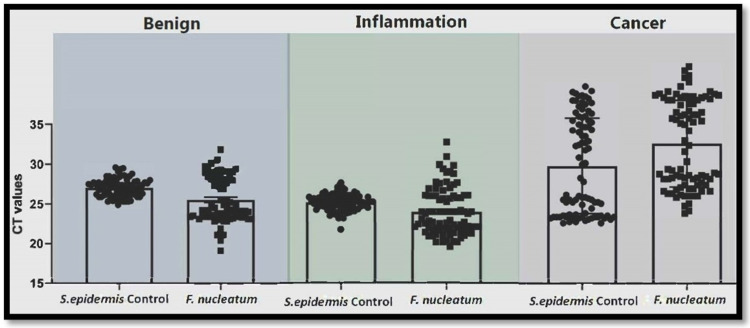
CT values of control S. epidermidis and F. nucleatum of all three areas, i.e., BPH, inflammation, and cancer BPH: benign prostatic hyperplasia
